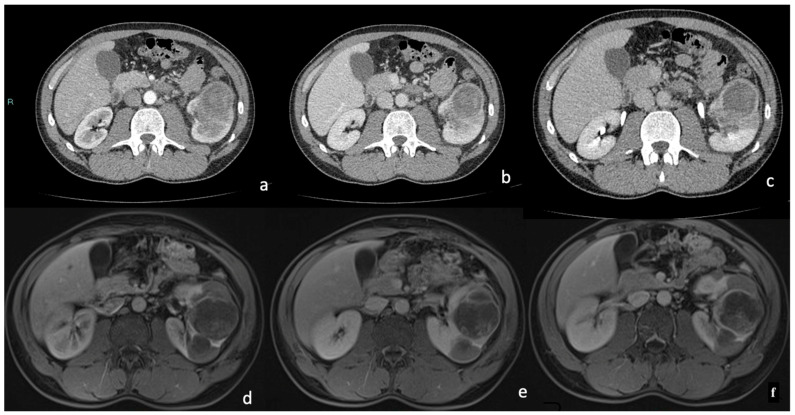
Enhanced CT in the arteriosus phase: (a) A hypodense renal lesion was detected on the anterior margin of the left kidney exophytic (<25%) with thick enhancing walls. (b) In the venous phase, and delayed phase (b,c) slightly intralesional enhancing was appreciable, with no clear detection of intralesional solid component. The lesion did not show significant washout, either centrally or peripherally. Enhanced MRI image in the arteriosus phase: (d) The left cortical renal mass appeared well-defined and hypointense. In the post-contrast image ((e), venous phase), irregular intralesional enhancing foci were present; in the delay phase (f), the lesion did not show considerable washout.
